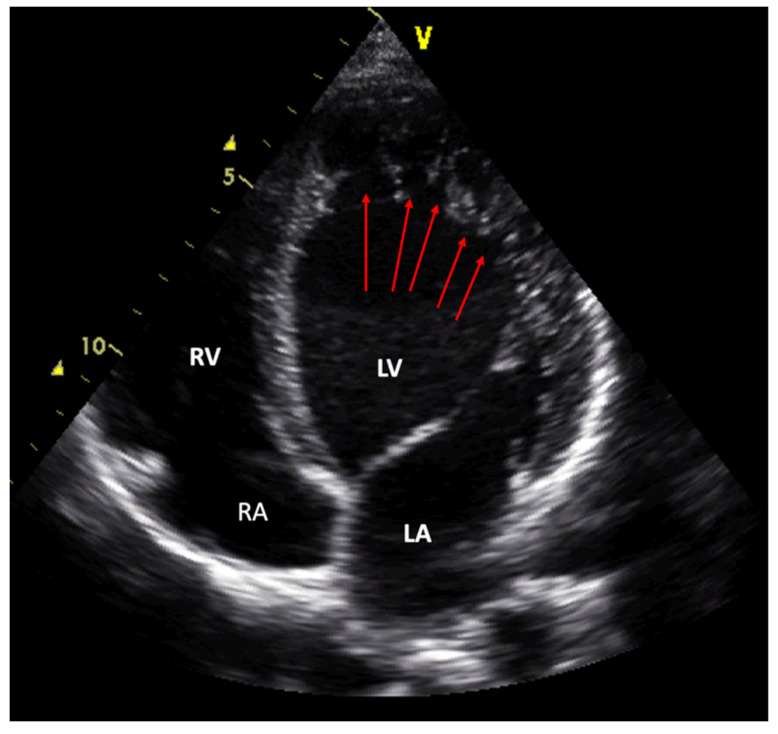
An echocardiographical apical 4-chamber view in a patient with NCCM. LV shows mild dilatation. The arrows mark the deep recesses in the noncompacted layer of the apical and lateral LV wall. LV—left ventricle; LA—left atrium; RV—right ventricle; RA—right atrium.
